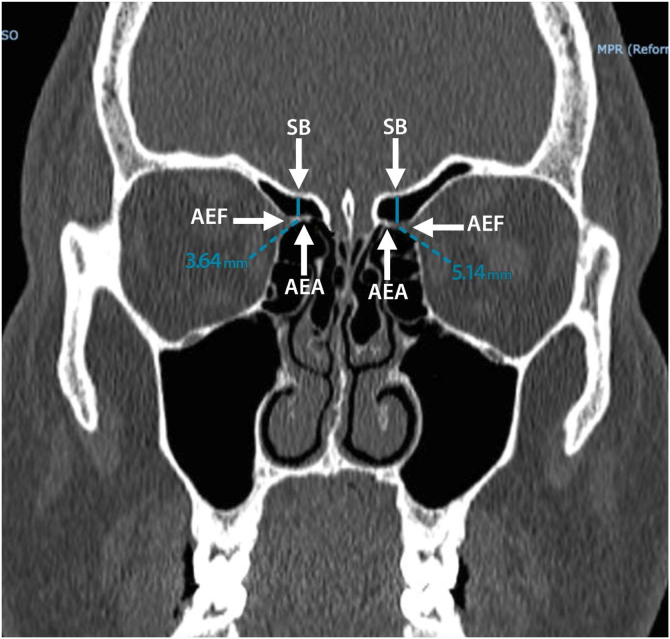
Computed tomography in coronal reconstruction showing the distance between the AEA and the SB. AEA, Anterior Ethmoidal Artery; SB, Skull Base; AEF, Anterior Ethmoidal Foramen.
